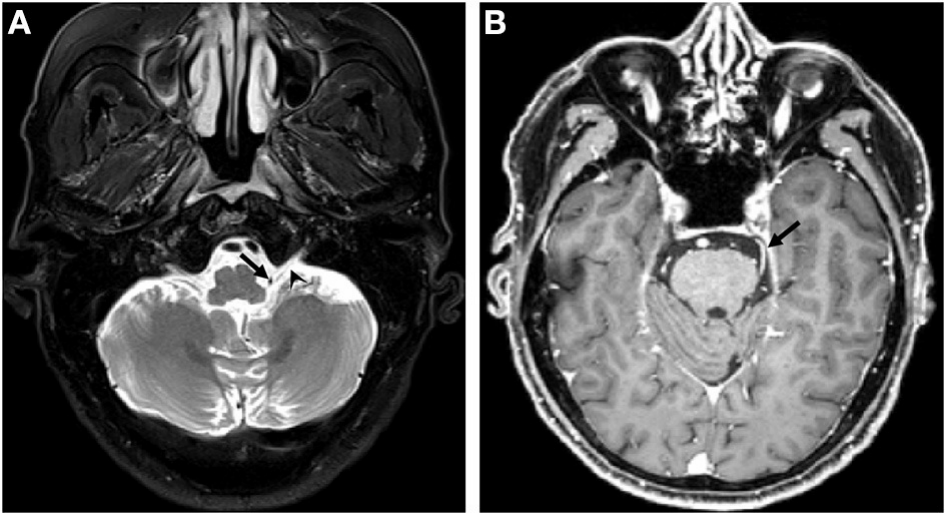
MRI before the first operation. (A) The posterior inferior cerebellar artery (arrow) touches the left glossopharyngeal nerve (arrowhead); (B) The SCA (arrow) compression of the left trigeminal nerve is not displayed clearly. SCA, superior cerebellar artery.
